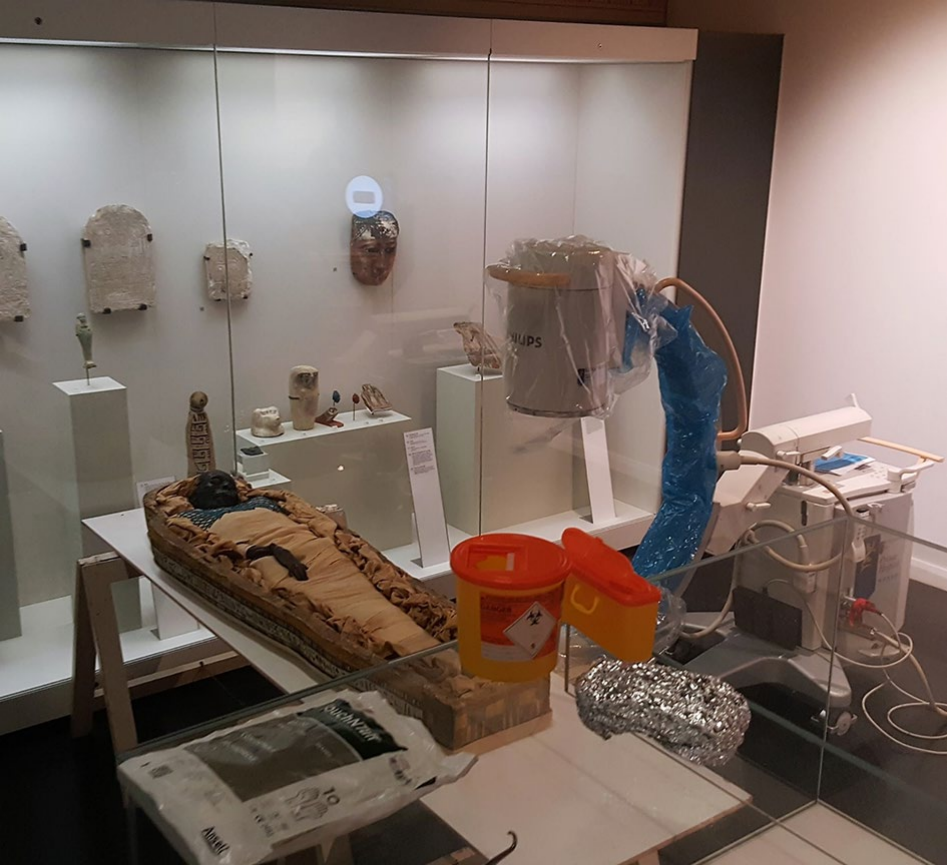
Sampling in situ. Ulster Museum, Belfast, Northern Ireland. Takabuti is positioned on the fibreboard and the X-ray imaging intensifier is positioned next to her. Copyright: Konstantina Drosou.
